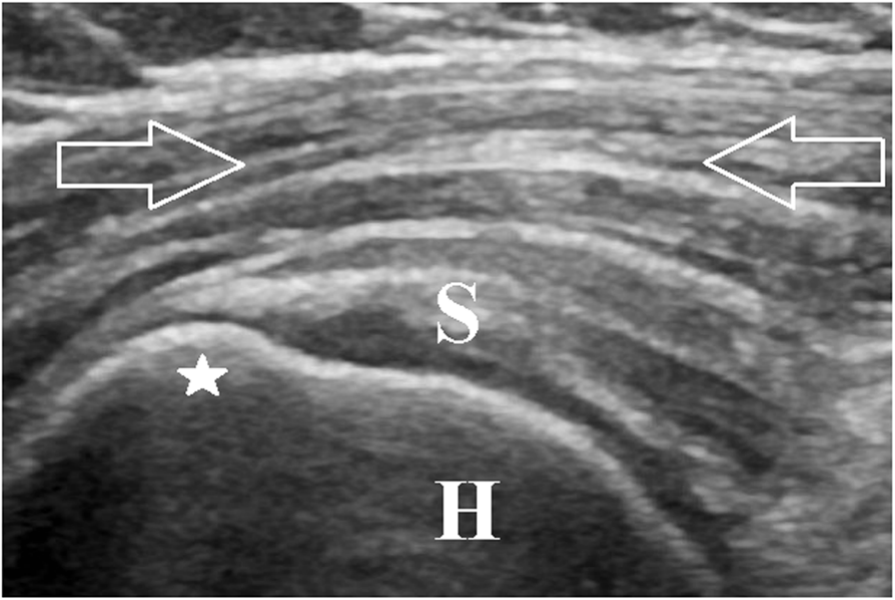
Ultrasound of a 47-year-old woman with type 2 diabetes mellitus. This long-axis sonographic image of the right deltoid muscle (open arrows) image is also obtained at the anterior aspect of the supraspinatus tendon (S), at its insertion at the greater tuberosity (solid star) of the proximal humerus (H). Notice the significant, diffusely hyperechoic (echogenic) appearance of the deltoid muscle. The patient had a body mass index of 32 kg/m2. The calculated ratio (deltoid muscle/humeral cortex) for this patient was equal to 0.67, consistent with a type 2 diabetes mellitus status
